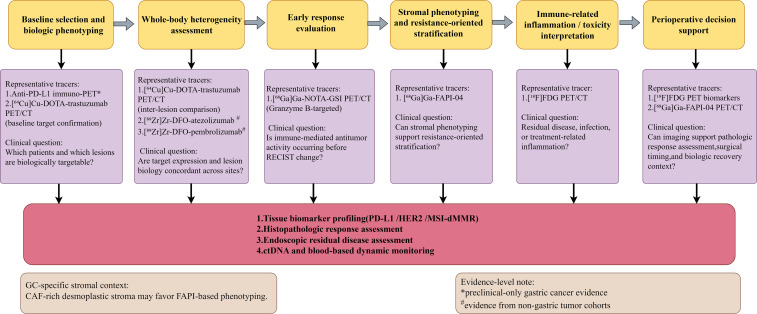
Proposed roles of molecular PET and immuno-PET across the gastric cancer immunotherapy pathway. CAF, cancer-associated fibroblast; ctDNA, circulating tumor DNA; FDG, fluorodeoxyglucose; FAPI, fibroblast activation protein inhibitor; GC, gastric cancer; RECIST, response evaluation criteria in solid tumors.
